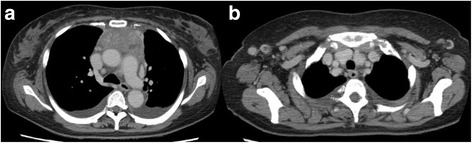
a Chest computed tomography demonstrating an anterior mediastinal mass measuring 65 × 35 mm, bilateral pleural effusion, and b mild peripheral lymphadenopathy
